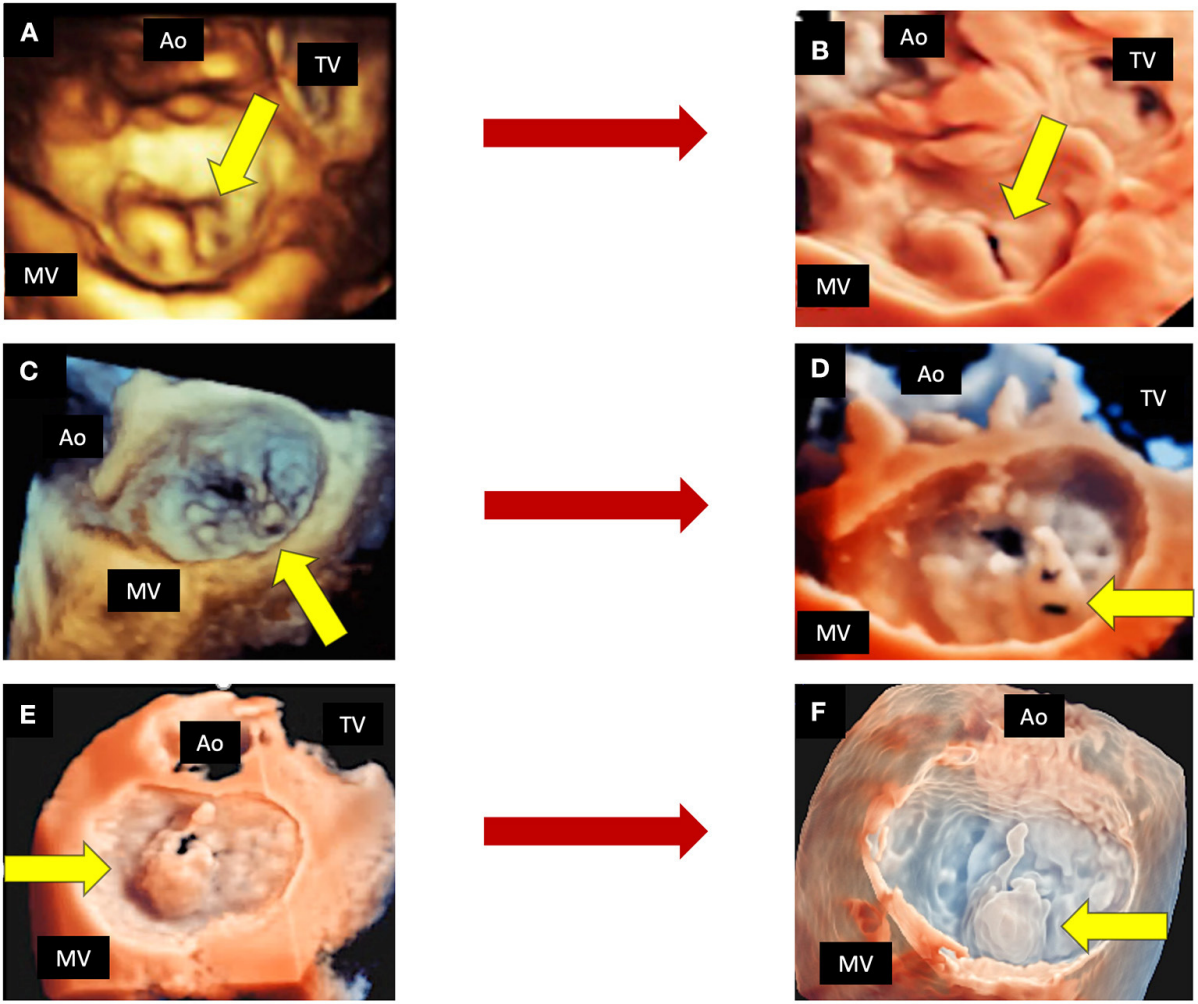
The role of new 3D tools in echocardiography. Shown are three examples of MVP, in which new tools may improve the quality of imaging. Top panels show a P2 prolapse by standard 3D TTE (A) and by TI rendering (B). Mid panels show a complex P2 prolapse by standard 3D TEE (C) and by TI rendering, which clearly shows the fragile texture of the leaflet (D). Bottom panels show a P2 flail by 3D TEE applying the TI tool (E) and the transparency effect (F). 3D, three-dimensional; Ao, aorta; MV, mitral valve; TEE, transesophageal echocardiography; TI, transillumination. TTE, transthoracic echocardiography; TV, tricuspid valve.
